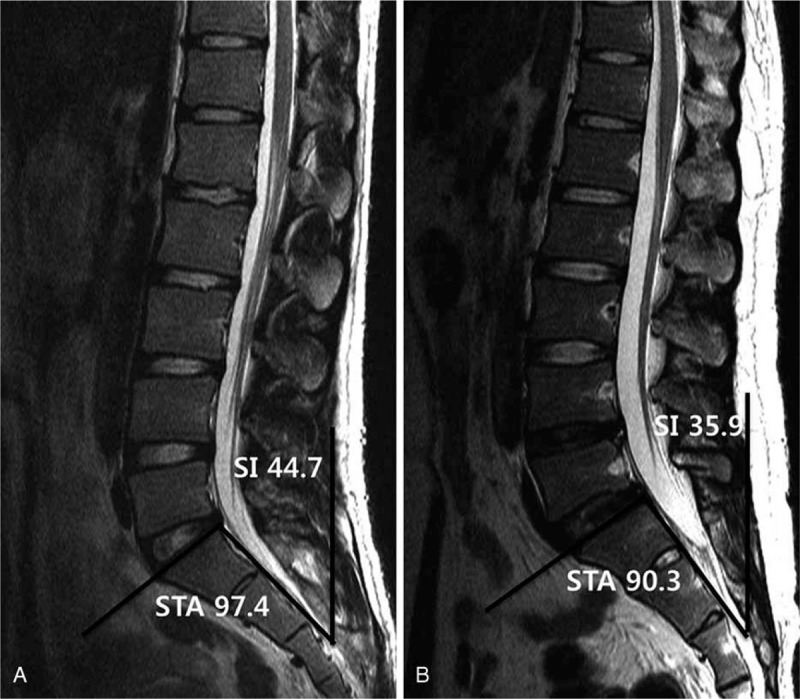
A, It denotes a magnetic resonance image (MRI) of a patient without disc degeneration and (B) shows an MRI of a patient with disc degeneration. The sacral inclination (SI) and sacral table angle (STA) are lower in a patient with disc degeneration (B) than in a patient without disc degeneration (A). The sacrum of the patient with disc degeneration is more vertical and showed more kyphosis (B) than that of the patient without disc degeneration (A).
